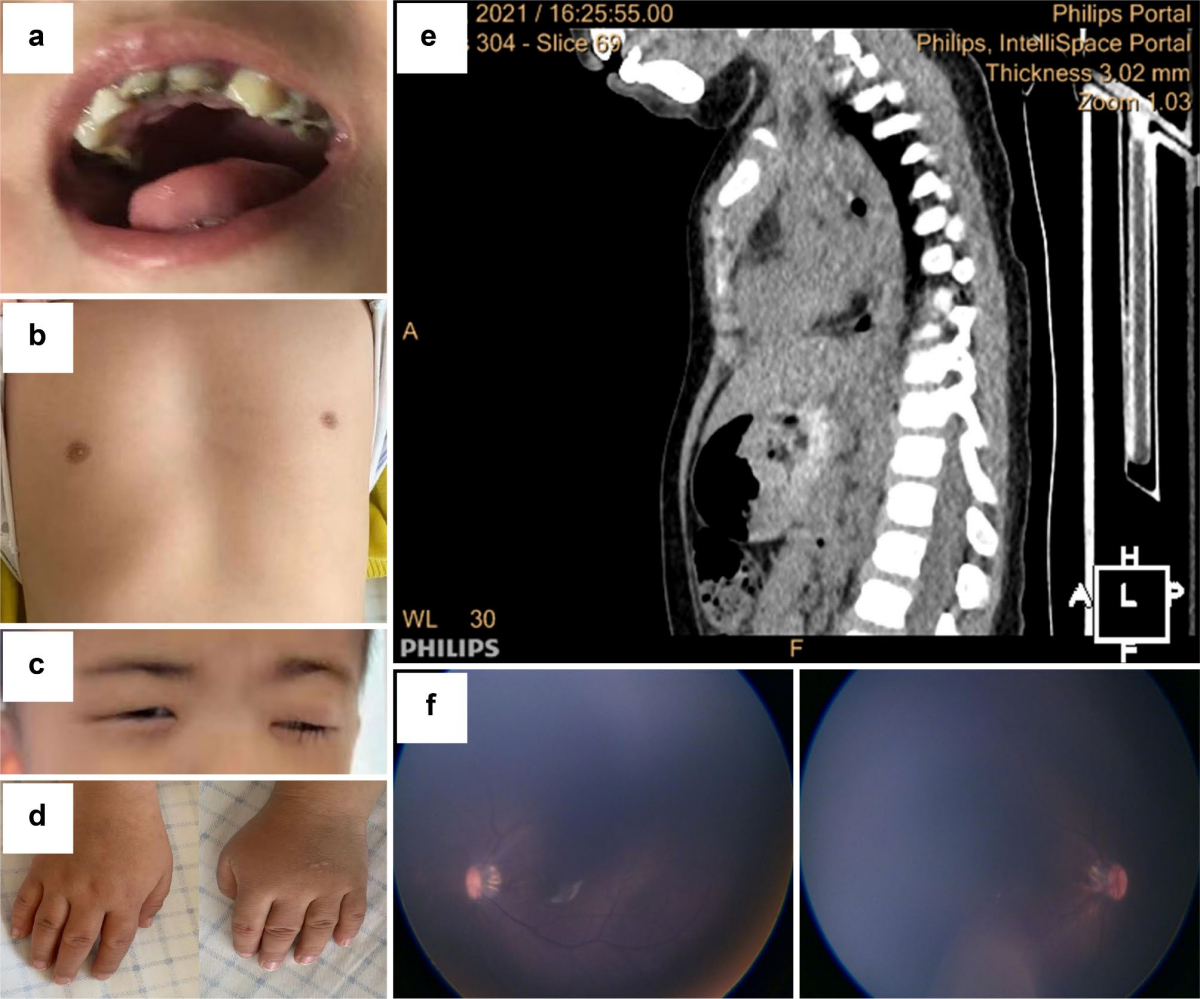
Characteristics of phenotypes. (a). The proband has deciduous teeth, showing bad enamel development with severe caries (7 teeth). (b) The proband shows malformations of the thorax and short ribs. (c) The proband has downward-slanted palpebral fissures and accompanying lower eyelid entropion and trichiasis. The eyelashes are longer on the right eye than on the left eye. (d) The proband has small hands, the fingers of which are short and contracted. (e) CT shows that the narrow thorax and the sternum’s lower half deform inwardly. (f) The proband shows bilateral optic nerve hypoplasia from the examination screening wide-field digital retina imaging system (Panocam)
